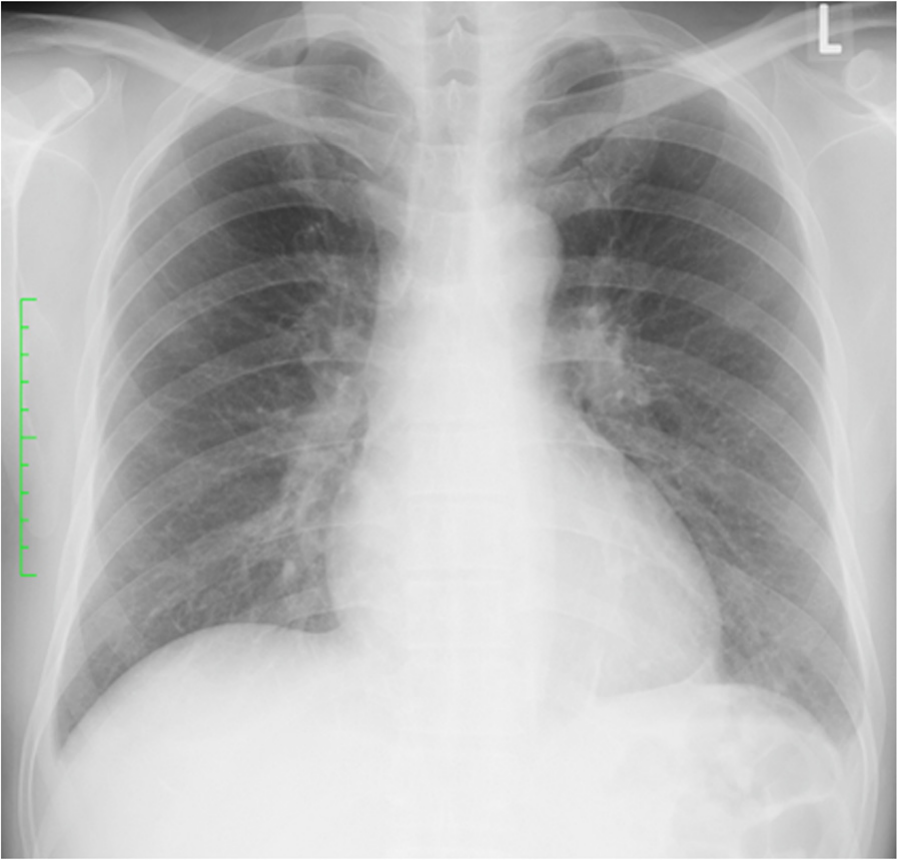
Chest radiograph, showing no infiltrative shadows
